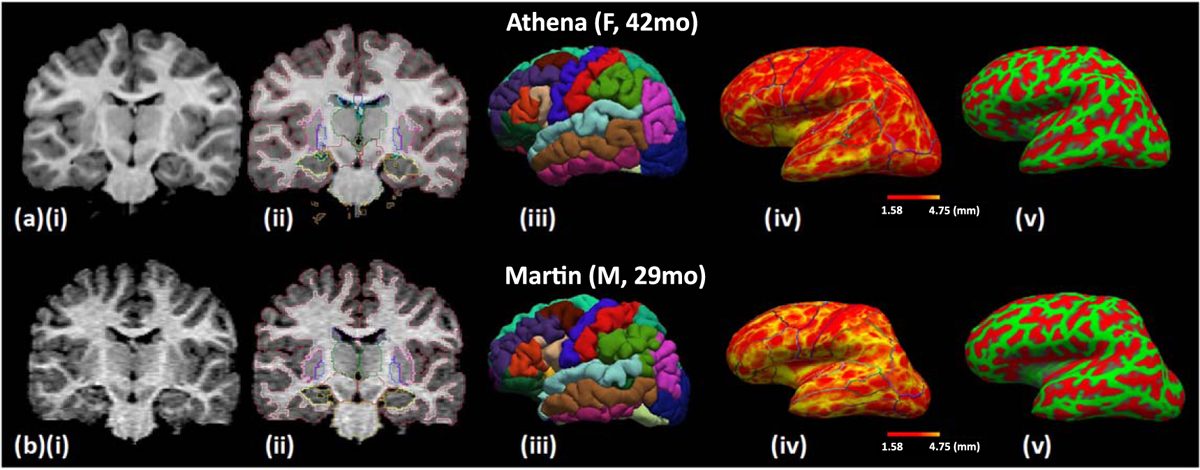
Brain automatic segmentation. (a) Brain segmentation of Athena a 42 months old (mo) female child (i) T1 brain MRI scan used for segmentation purposes. (ii) Automated tool outlining the brain anatomical structures based on established anatomical atlas (Desikan-Killiany). (iii) 3D reconstruction of the brain with each color indicating a different anatomical region following the same anatomical atlas. (iv) Gray matter thickness maps with the red area corresponding to lower and the yellow to higher thickness (mm). (v) Curvature map as an index of brain folding with green color corresponding to gyri and red color to brain sulci. (b) The same process was followed the male model (Martin 29 mo) for comparison purposes.
